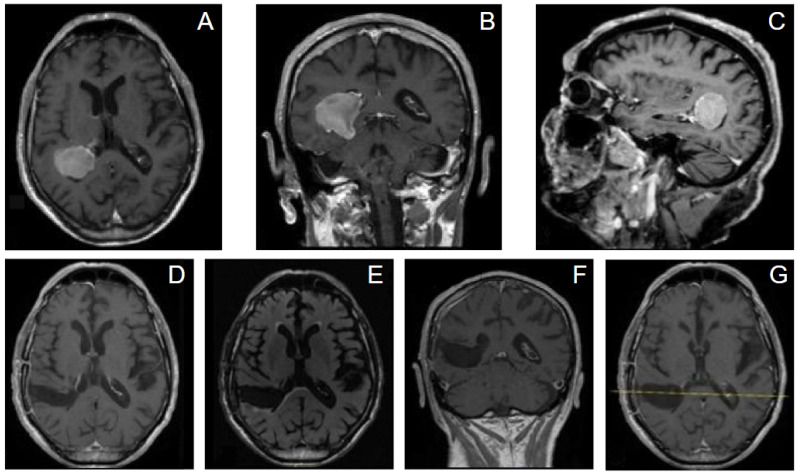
(A–C) The axial (A), coronal (B) and sagittal (C) contrast-enhanced, preoperative MRI showed a heterogeneously enhancing mass arising from the trigone of the right lateral ventricle. (D,E) The axial contrast enhanced postoperative MRI (D) revealed a complete tumor resection with minimal FLAIR changes (E) demonstrating no damage to the brain immediate surrounding the tubular trajectory. (F,G) At 3 months from surgery there is no evidence of tumor recurrence and/or evidence of brain injury.
